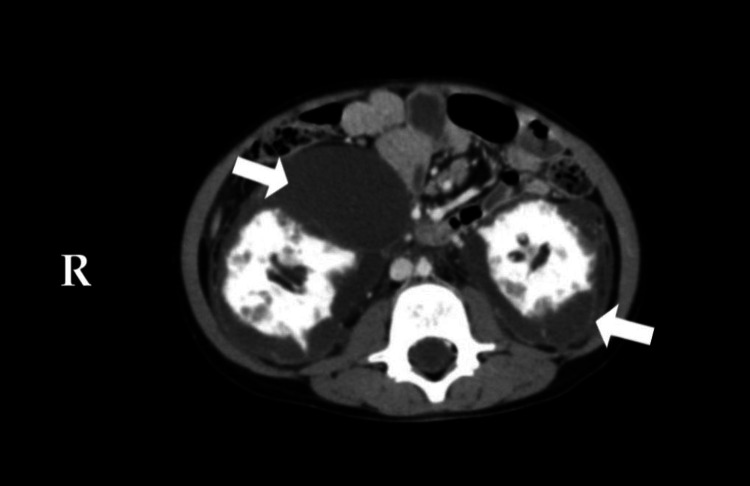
Contrast-enhanced CT of the abdomen (axial views): there are bilateral non-enhancing perinephric collections (white arrows). Both kidneys have normal perfusion
